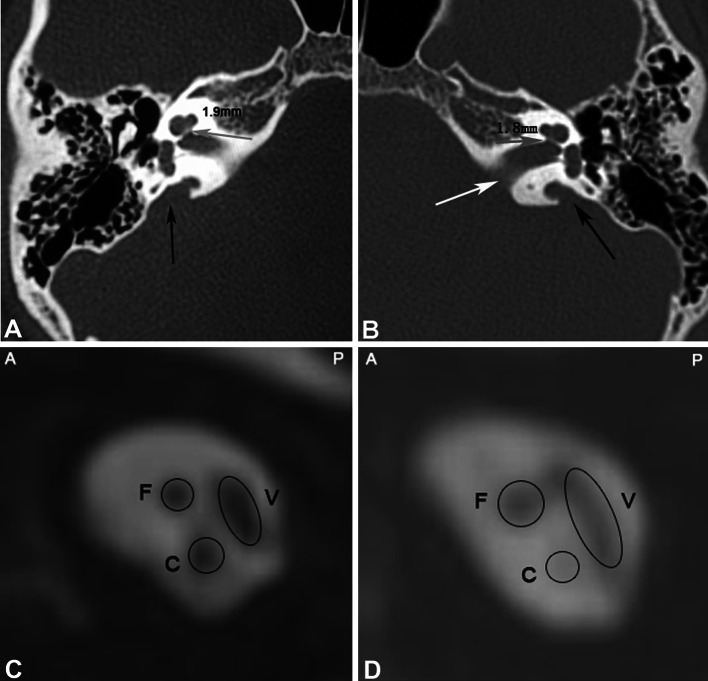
Enlargement of bilateral IAMs with CN hypoplasia. Male, 6 years old. Bilateral severe hearing loss. a, b Enlargement of the IAM, binaural enlarged vestibular aqueducts. The CNC on the right side is 1.9 mm (a), whereas 1.8 mm on the left side (b). c, d Oblique sagittal reconstruction of MRI imaging shows normal development of the right CN, FN and vestibular nerve in the IAM (c); the diameter of the left CN is smaller than that of the FN, suggesting CN hypoplasia (d)
